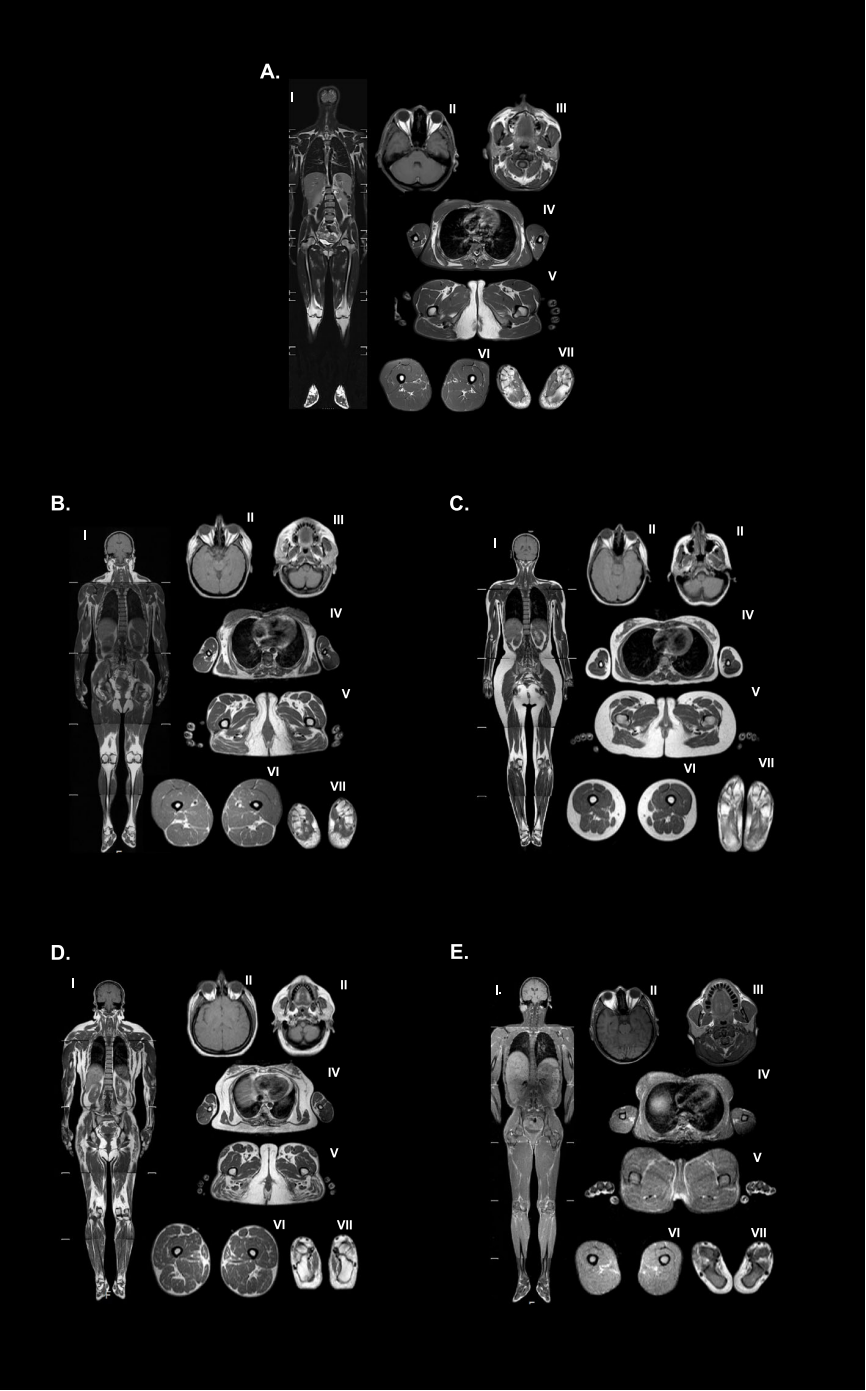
Comparison of fat distribution. The whole-body MRI confirms generalized fat loss in the patient who had a homozygous pathogenic variant in the *LMNA* gene. Adipose tissue is well preserved around mons pubis and external genital region similar to heterozygous *LMNA* R582H patient and typical FPLD2 patients while fat tissue loss is noted in a generalized pattern in the scalp, mammary gland, abdomen visceral/subcutaneous, and extremities. Fluid like signal is detected in the bone marrow. Supraclavicular subcutaneous fat was preserved, but the amount of fat was significantly decreased in contrast to heterozygous *LMNA* R582H and typical FLPD2. The liver was steatotic and diffusely enlarged. Fat loss is partial in heterozygous *LMNA* p.R582H carrier (Fig. 2b) affecting the limbs, abdomen, breasts and the lower part of the body which is similar to typical FPLD2, although more subcutaneous fat was observed in the upper part of the trunk, over the shoulders, and head and neck (Fig. 2b and d). Retroorbital fat is preserved in all patients. a: Fat distribution in the patient with homozygous *LMNA* pathogenic variant, R582H; b: Fat distribution in the patient with heterozygous *LMNA* pathogenic variant, p.R582H; c: Fat distribution in a healthy control (28 years old, female); d: Fat distribution in a 30-year-old female with typical FPLD2 caused by heterozygous *LMNA* pathogenic variant p.R482W (c.1444C > T); e: Fat distribution in a 30-year-old female with the classical CGL1 phenotype caused by homozygous *AGPAT2* pathogenic variant p.C48X (c.144C > A). In each panel I. Whole-body T1-weighted imaging; II. Retroorbital, axial T1 weighted- imaging; III. Head and neck, axial T1 weighted-imaging; IV. Trunk, axial T1 weighted-imaging; V. Pelvic region, axial T1 weighted-imaging, VI. Upper leg, axial T1 weighted imaging; VII. Sole, axial T1-weighted imaging
